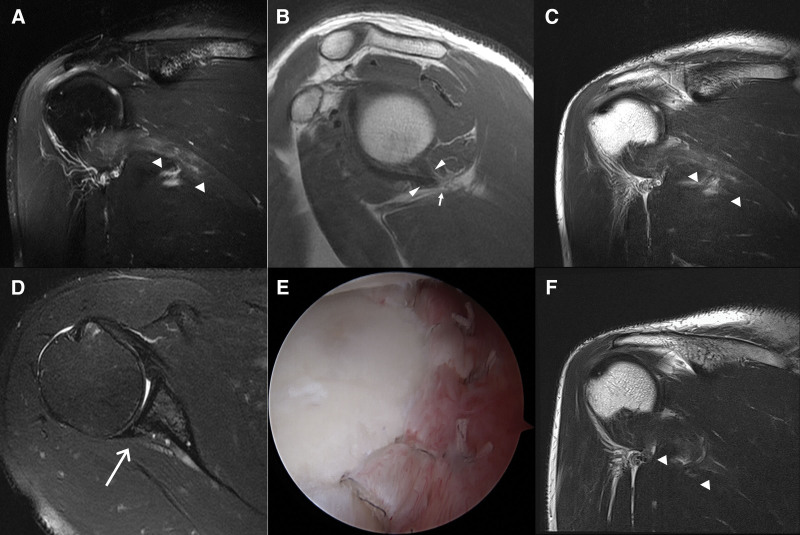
Preoperative and postoperative MRI images of a 42-year-old male patient with right shoulder vague pain. The patient’s shoulder pain began 5 years ago, and the pain aggravated 1 month ago with night pain. Physical examination reveals full passive ROM, positive abduction and external rotation provocation test, and tenderness over the quadrilateral space. The patient underwent arthroscopic labral repair, and the symptoms significantly improved by the 4-week follow-up visit. A. Preoperative fat-saturated T2-weighted (FST2W) oblique coronal image demonstrates denervation edema of the teres minor (short arrowheads) and a small subacromial-subdeltoid bursitis. B. Oblique sagittal T1-weighted image (T1WI) reveals decreased volume of the teres minor and the posteroinferior joint capsule tethered (long arrowheads) to the axillary neurovascular bundle (short arrow). C. Preoperative T2-weighted (T2W) oblique coronal image displays denervation edema mixed with fatty infiltration. D. Preoperative FST2W axial image exhibits a posterior labrocapsular complex tear (long arrow). E. Arthroscopic image presents satisfactory labral repair. F. Postoperative T2-weighted oblique coronal image obtained 4 weeks after the arthroscopic surgery shows decreased high signal intensity of the teres minor compared to that of Figure C.
